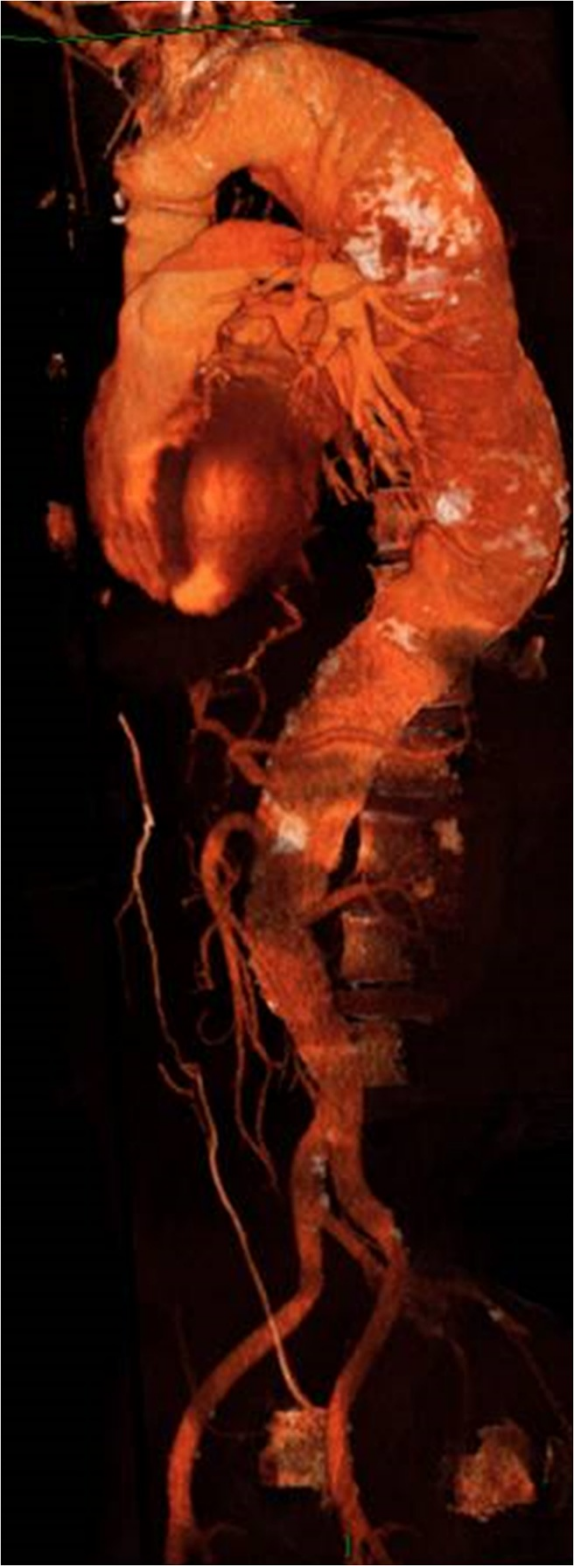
A CT reconstruction of a type II TAAA.
